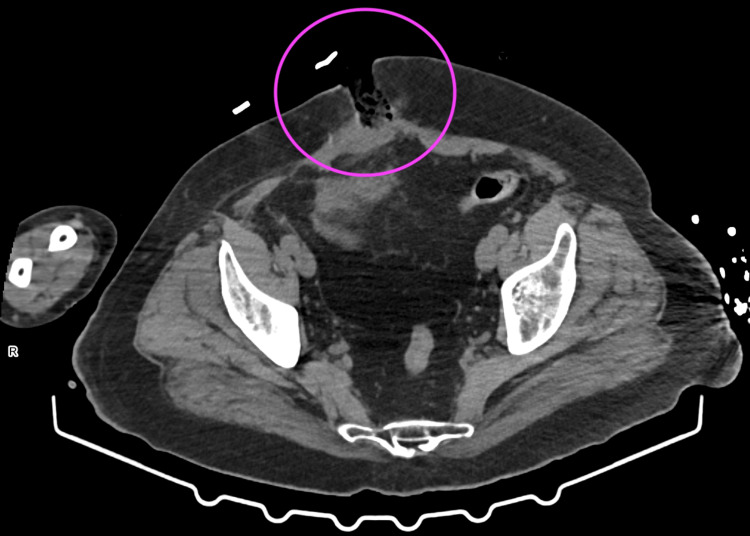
CT image of evisceration Disruption of the continuity of the right rectus abdominis muscle over a length of 25 mm with protruding adipose tissue (pink circle) - hernia with a likely incarcerated loop of intestine with a collapsed lumen.
